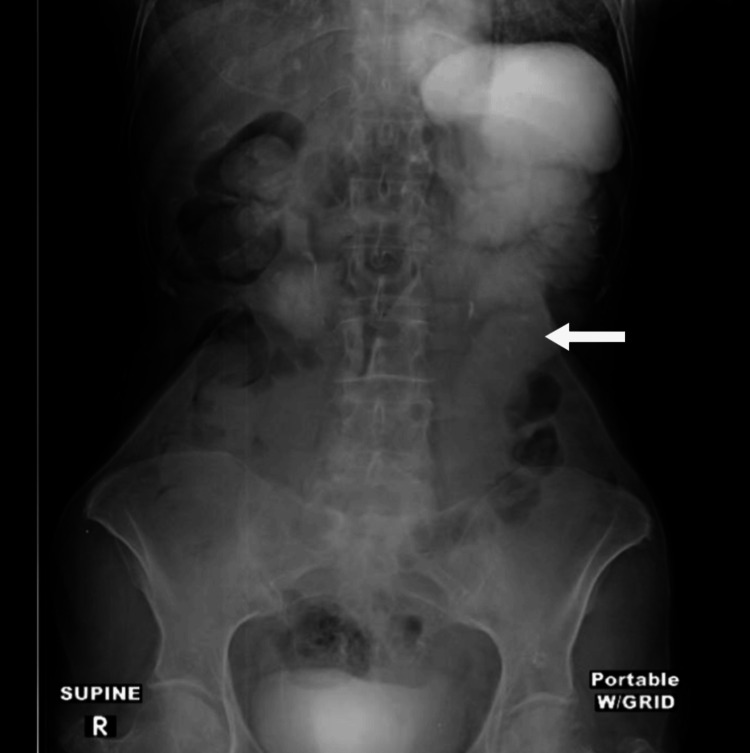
Abdominal X-Ray without contrast. The oral contrast from the previous day had only progressed into the proximal segments of the jejunum (white arrow), which were noted to be mildly distended.
